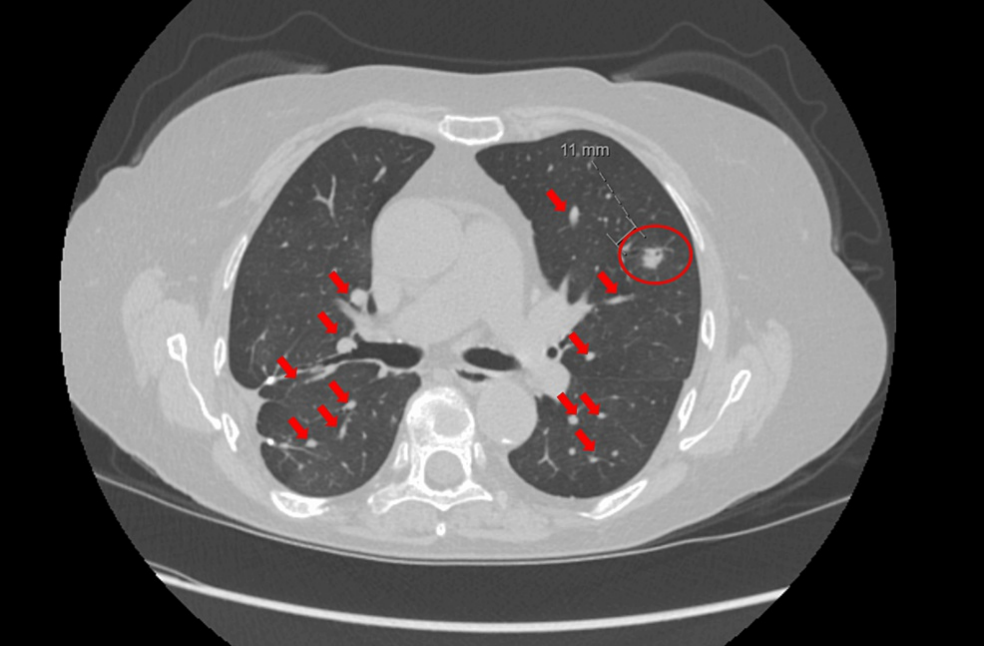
CT chest showing 11 mm left lung carcinoid tumor (circle) with multiple smaller carcinoid tumorlets (arrows)
